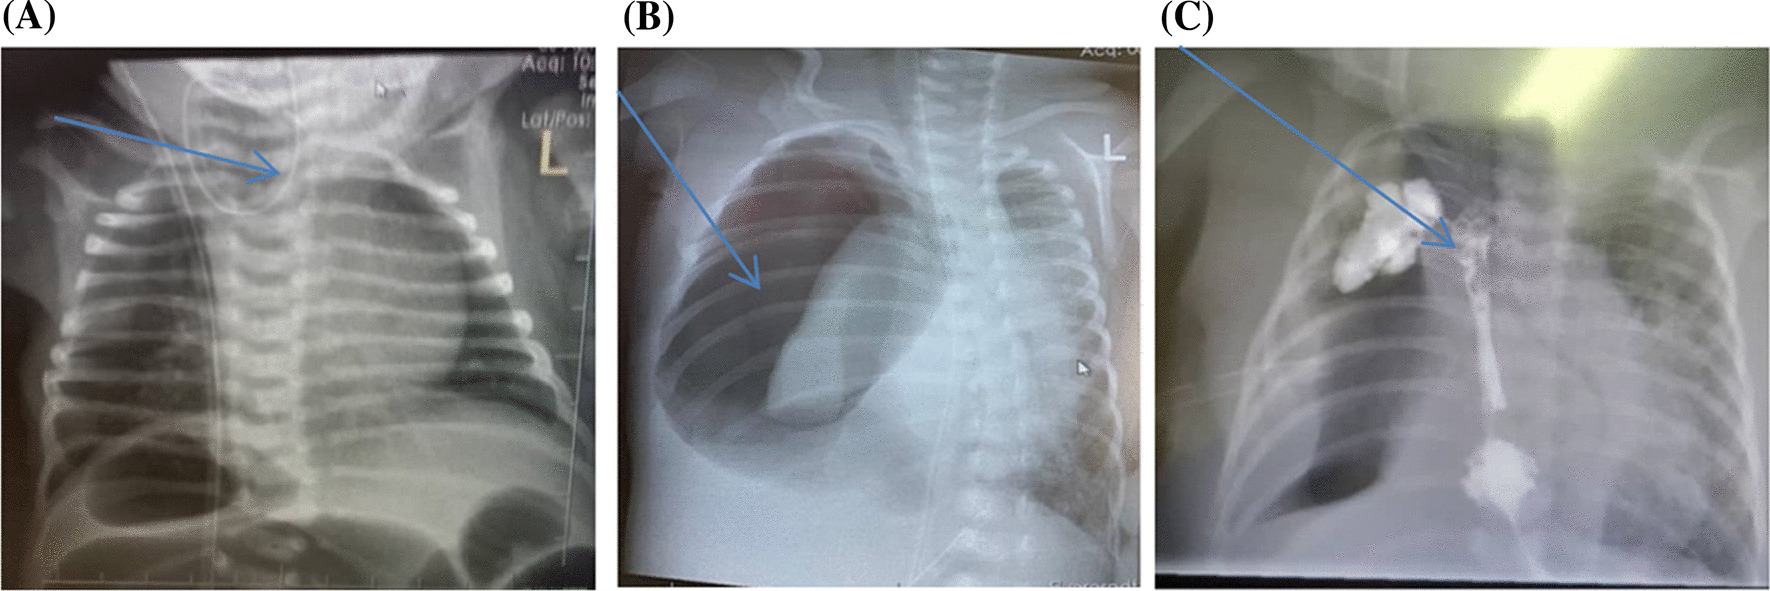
A Chest X-ray showing coiling of nasogastric tube, blue arrow shows coiling of nasogastric tube in upper esophageal pouch. B Chest X-ray showing pneumothorax, blue arrow shows right tension pneumothorax, collapse of ipsilateral lung and contalateral shift of the mediastinum. C Chest X-ray showing anastomotic leakage, blue arrtow shows leakage of the gastrografin swallow into the right lung
